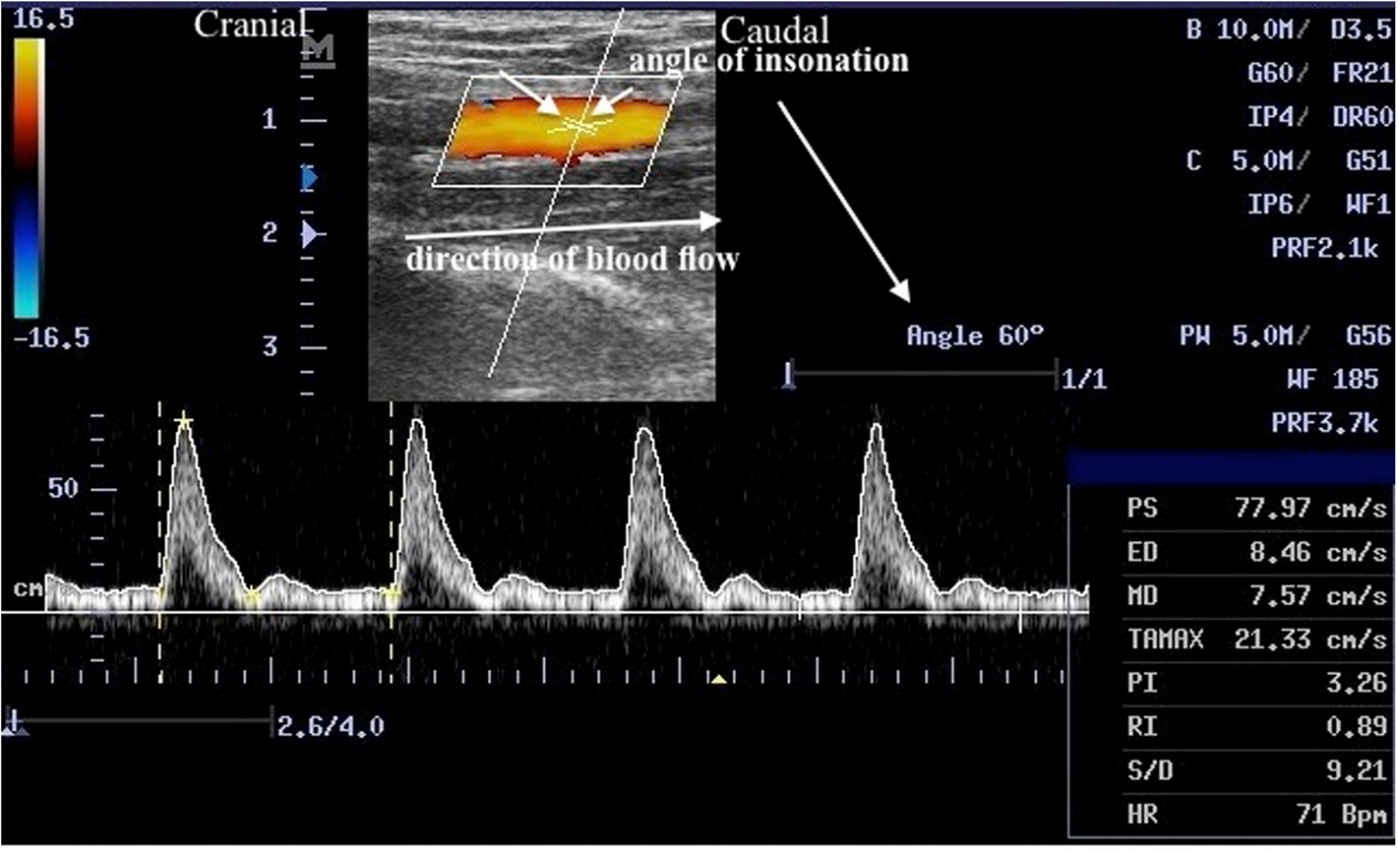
PWD Ultrasound of the brachial artery after brachial plexus block. PS: peak systolic velocity (cm/s.). ED: end-diastolic velocity (cm/s.). TAMAX: time average maximum velocity (cm/s.)
